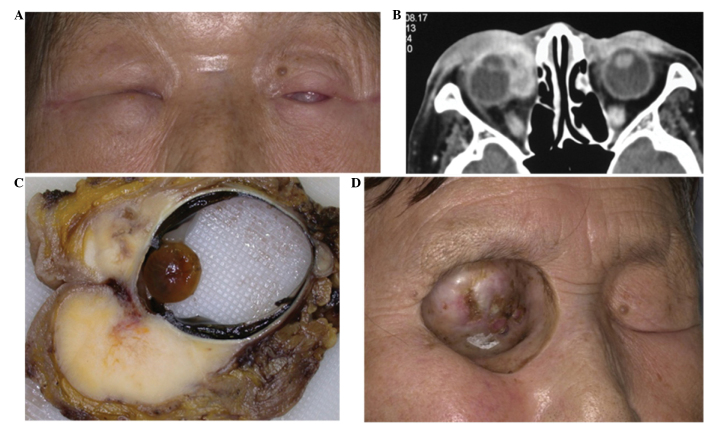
(A) Facial photograph and (B) computed tomography prior to treatment; (C) gross appearance of tumor following orbital exenteration; and (D) the patient following treatment.
